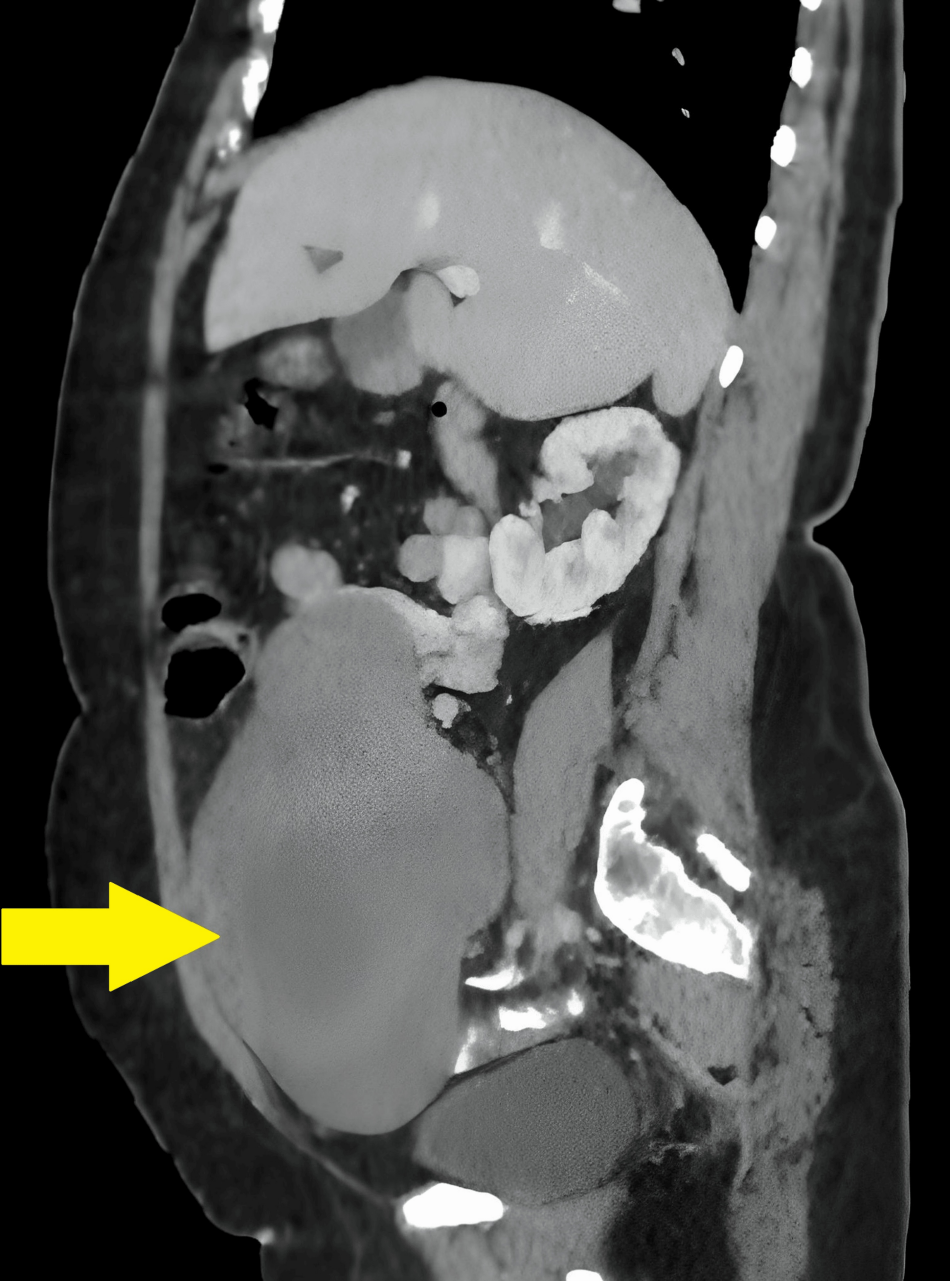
CECT of the abdomen showing the tumor mass (yellow arrow) CECT: contrast-enhanced computed tomography
